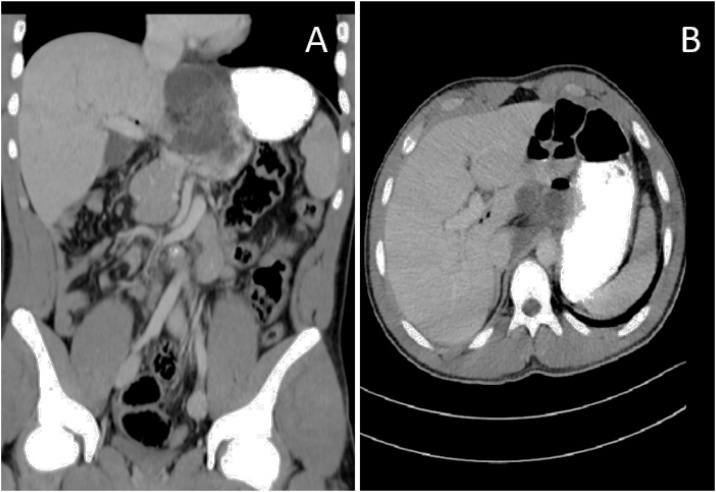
Abdominal computed tomography (CT). (A) Coronal view showing residual contrast material in the stomach, malrotation of the abdomen on the mesenteroaxial axis and air bubbles are found in the falciform ligament. (B) axial section with air bubbles shown in the falciform ligament.
